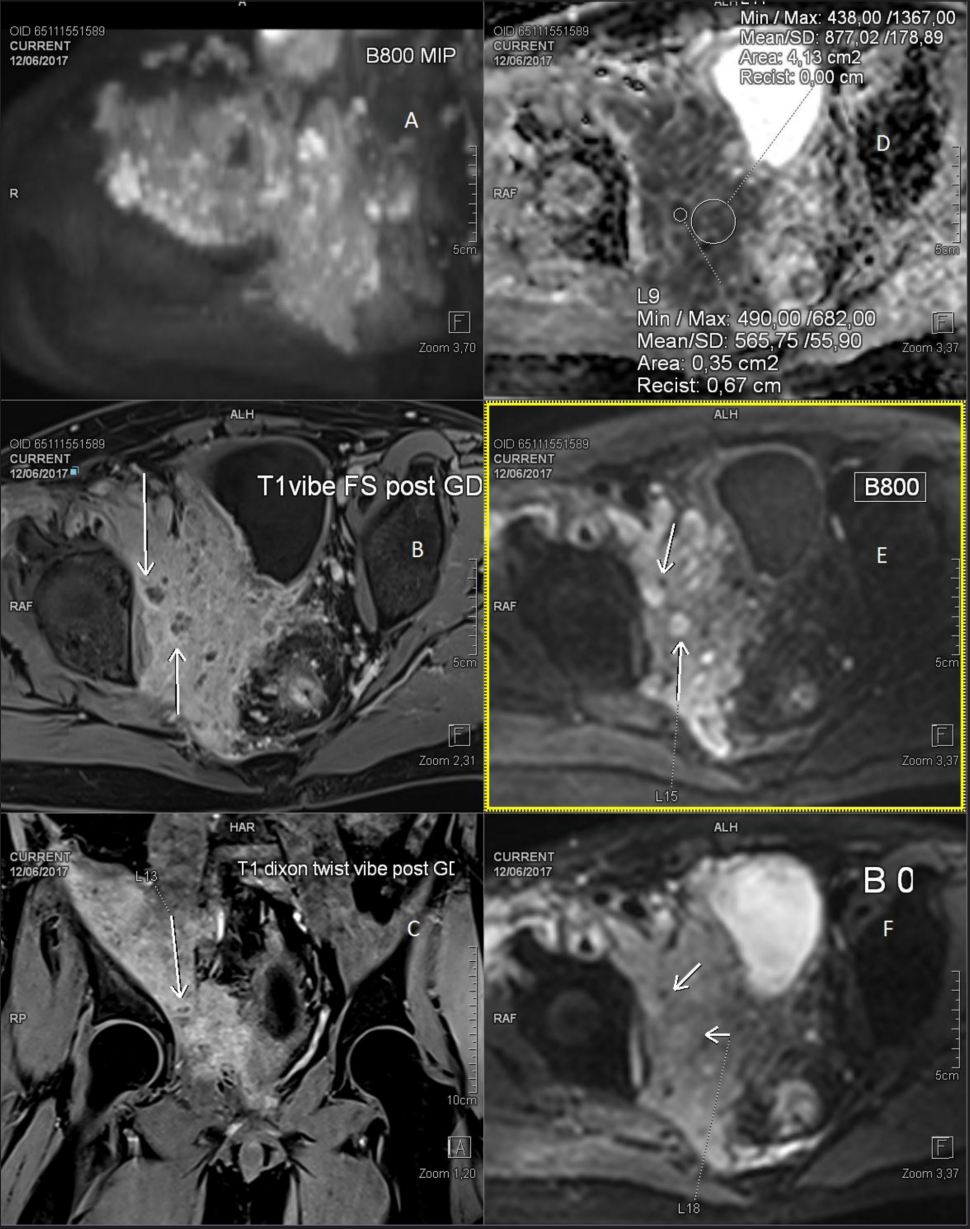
MRI at initial presentation with T1 fatsat images post gado (B, C) and DWI images (A, D, E, F).
